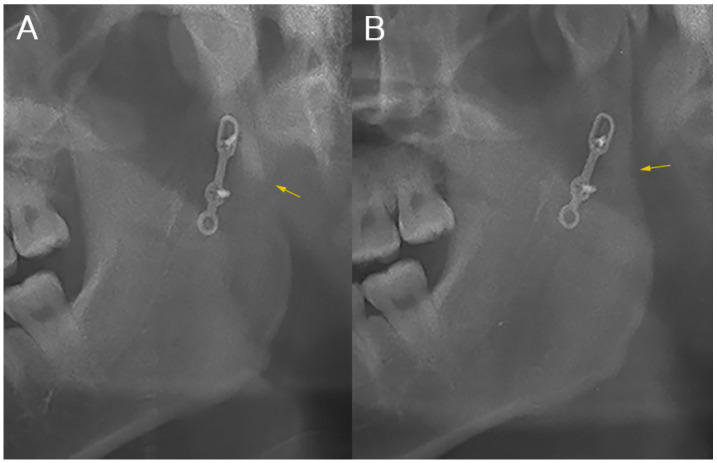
A clinical case in which anterior angulation of the condylar process was normalized at postoperative 6 months. (A) In the panoramic radiograph taken at postoperative day 1, posterior ramal bony step remained (arrow) although the condylar reduction was completed; (B) In the panoramic radiograph taken at postoperative 6 months, continuous posterior border of condyle can be seen (arrow).
